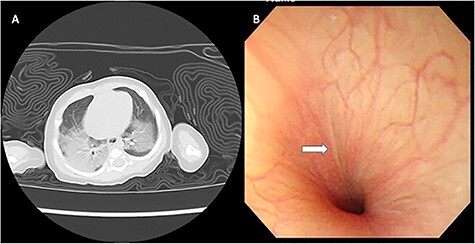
Bilateral centrilobular opacified lesions detected by chest computed tomography (A). Upper gastrointestinal endoscopy shows a longitudinal linear scar at the gastroesophageal junction (B).
